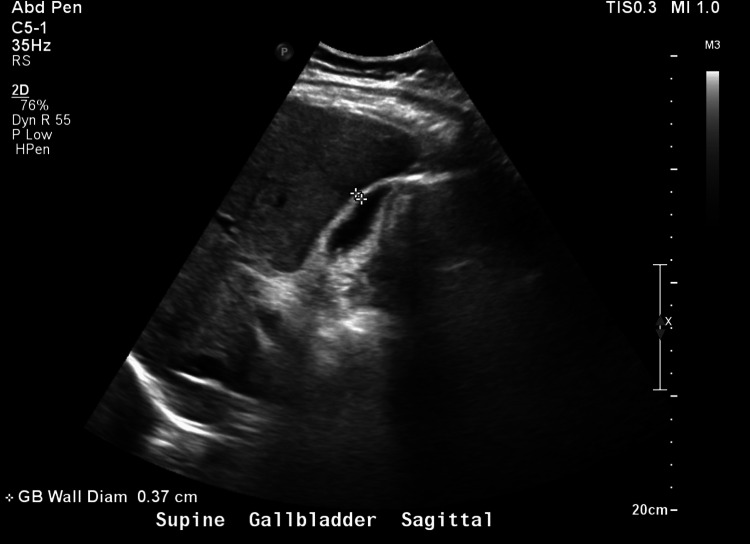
Ultrasound Abdomen Right Upper Quadrant - Gallbladder
